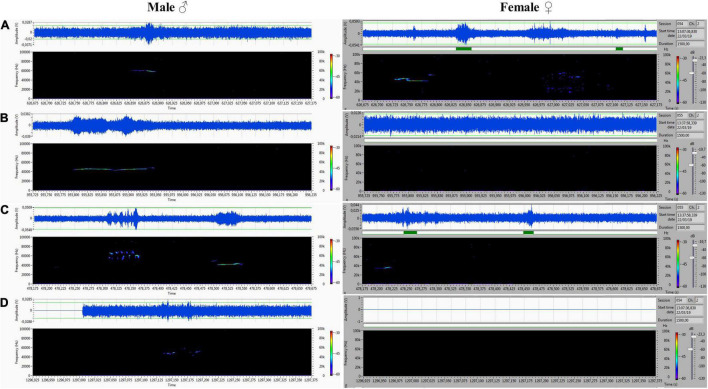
(A–D) Various forms included flat-type, multistep, and step down on the male’s side On the female’s side, the ultrasonic spectrum is distinctly different. All ultrasounds can be unambiguously assigned to the emitter.
